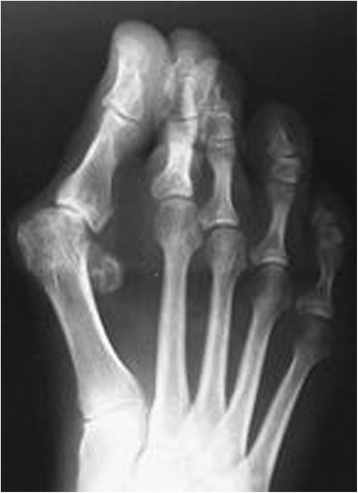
Survey Case 2: Dorsoplantar weight-bearing radiograph of a patient’s right foot. Complaints related to her hallux valgus deformity lasting since 2 years. She favours surgical treatment as conservative measures had failed
